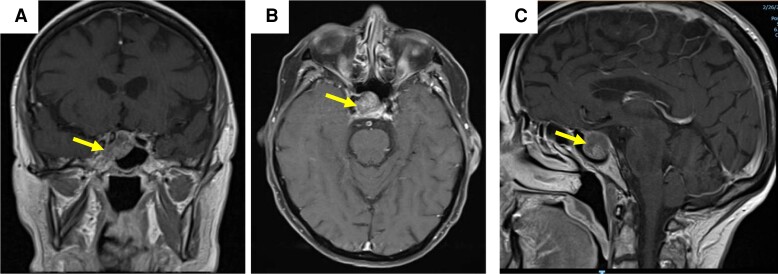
T1-weighted magnetic resonance imaging of the brain from case 2. Arrows point to the mass in all images. A, Coronal section of partially cystic sellar and suprasellar mass causing mass effect on the optic chiasm. The mass is medial to the right cavernous sinus. B, Sagittal cut showing the mass abutting the optic chiasm in the sella. C, Axial cut showing a well circumscribed mass in the sella.
